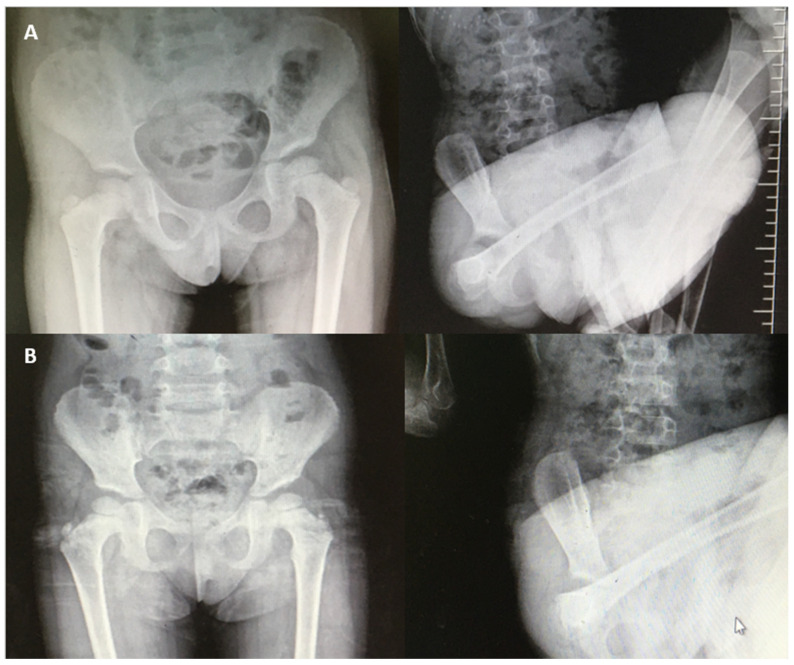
(A) Radiographs showing normal and dislocated hip positions in a 46-month-old female patient. (B) Radiographs showing normal and dislocated hip positions in a 54-month-old female patient.
